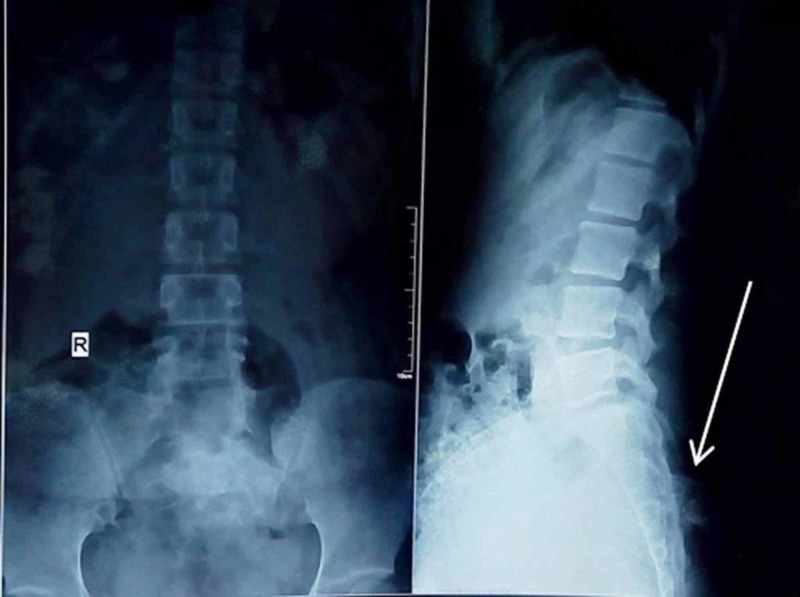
Radiograph showing a bony growth arising from the S2-3 level
